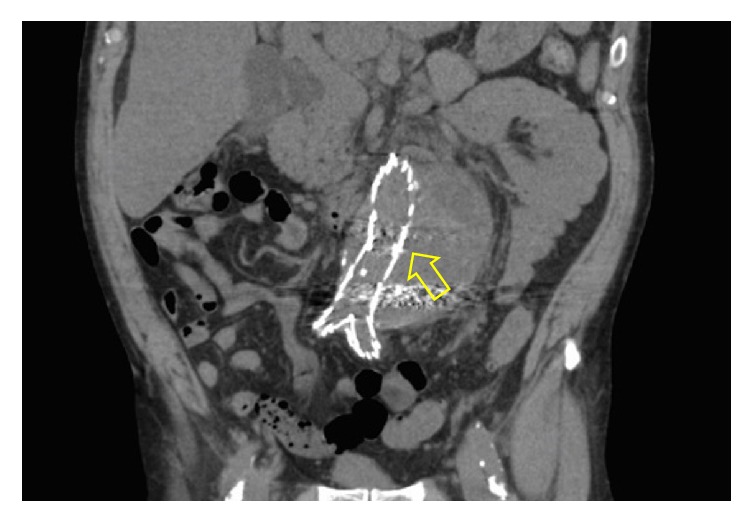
CT abdomen showing an expanding abdominal aortic aneurysm with few gas bubbles around prior endovascular graft (arrow).
